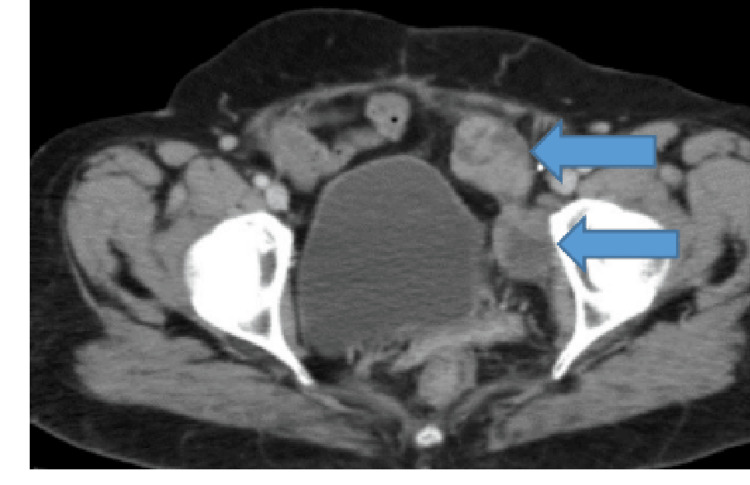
CT scan axial view showing heterogenous masses along the left iliac vessels suggestive of recurrent disease in the left hemi pelvis.
